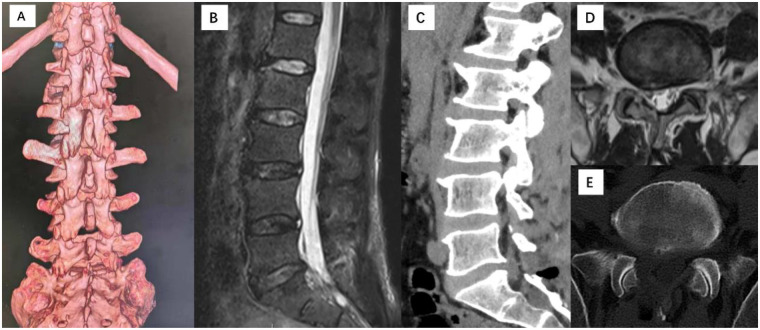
Postoperative imaging follow-up assessment. (A) Three-dimensional CT images demonstrate minimal bone removal from the superior edge of the right S1 lamina and the medial margin of the facet joint, with preservation of the bone at the inferior edge of the L5 lamina. (B,C,D,E) MRI and CT images confirm successful removal of the herniated nucleus pulposus and complete decompression of the spinal cord and nerve roots.
